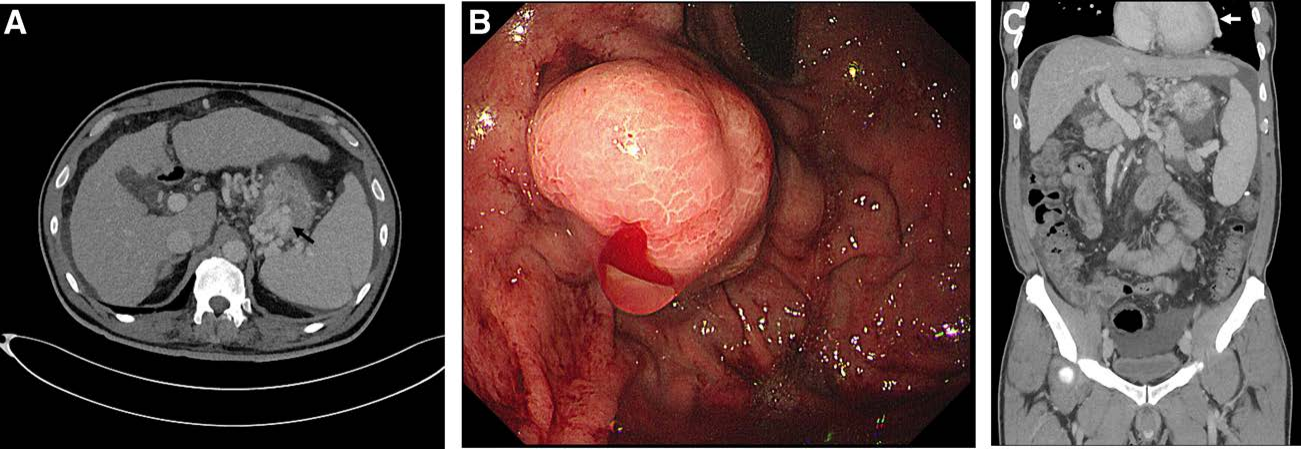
(A) CT image showing a tortuous and enlarged gastric varix (arrow) in the gastric fundus. (B) Endoscopic image of the gastric varix with bleeding appearing as a polypoid submucosal mass. (C) Coronal CT image of the pericardial vein (white arrow) running along the left border of the heart. CT = computed tomography.
